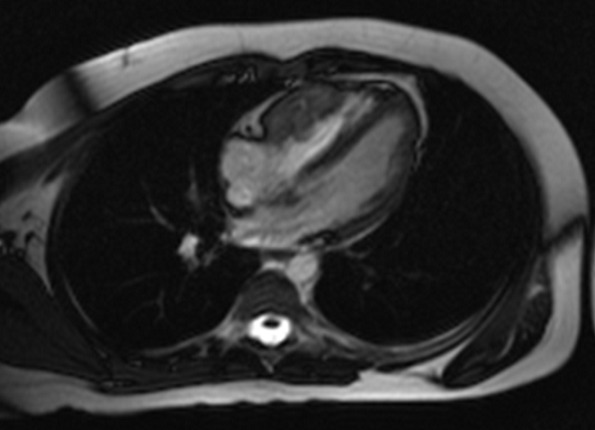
Cardiac magnetic resonance image, heart four chambers view showing right ventricle thrombus (arrow)
